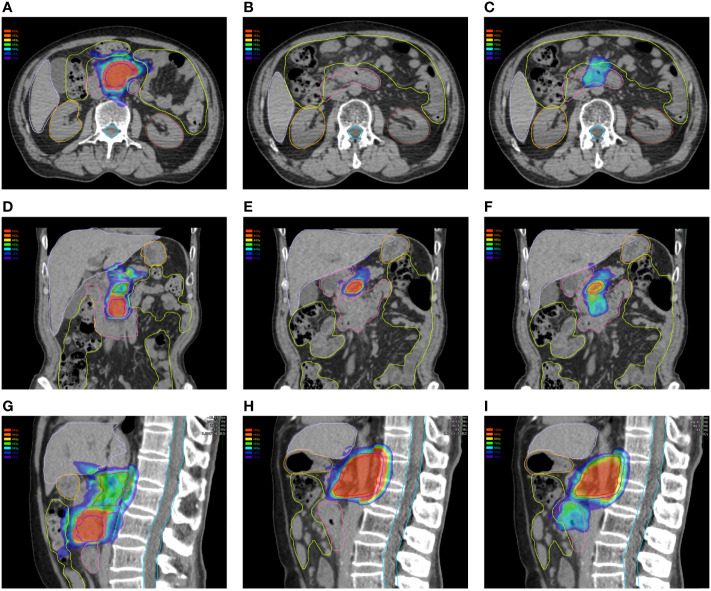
Dose distributions of the first plan in the (A) horizontal plane, (D) coronal plane, and (G) sagittal plane. Dose distributions of the first plan in the (B) horizontal plane, (E) coronal plane, and (H) sagittal plane. Dose distributions of the first plan projected to the second CT scans in the (C) horizontal plane, (F) coronal plane, and (I) sagittal plane.
